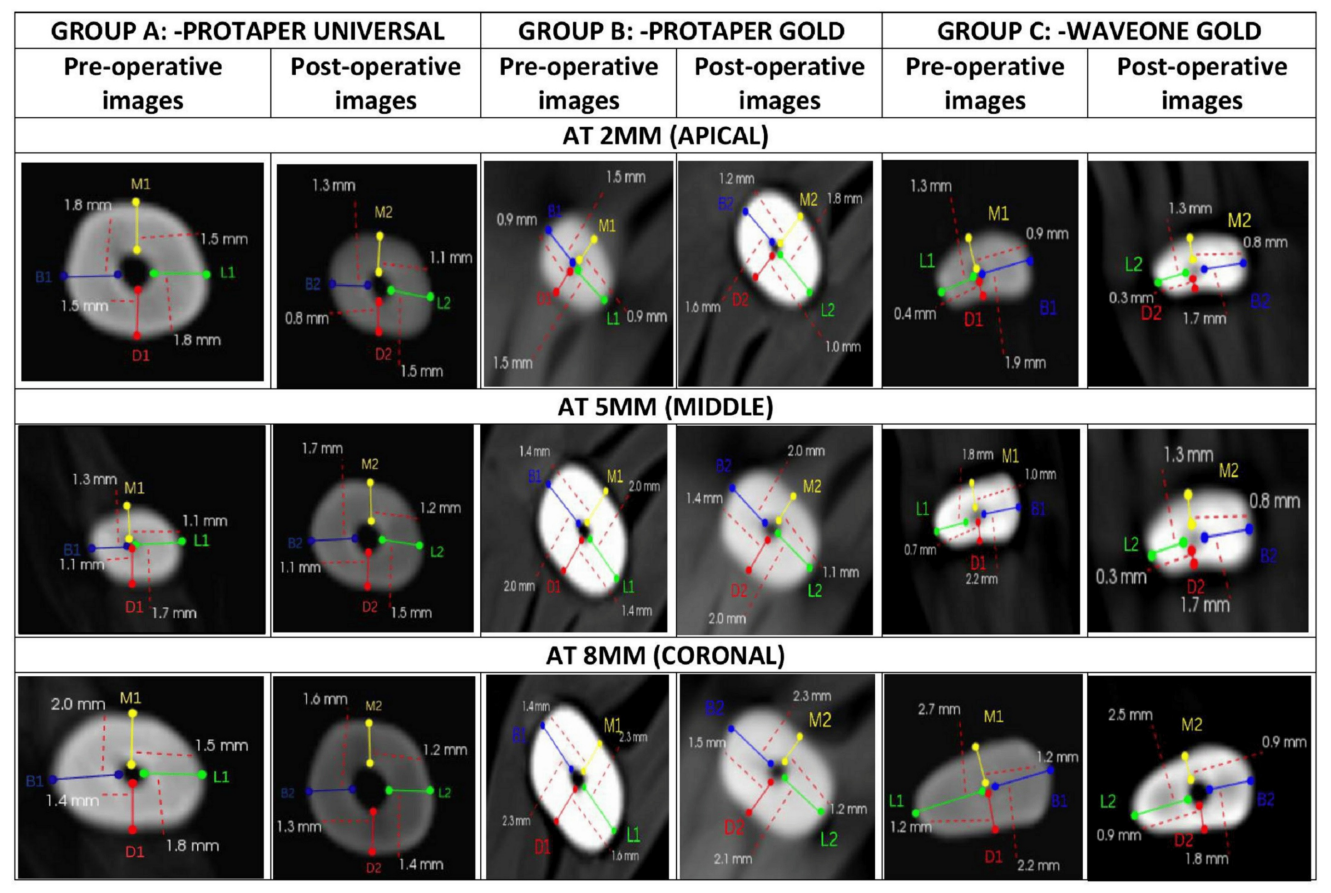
Pre and post-operative images of tooth depicting remaining dentin thickness using cone-beam computed tomography
